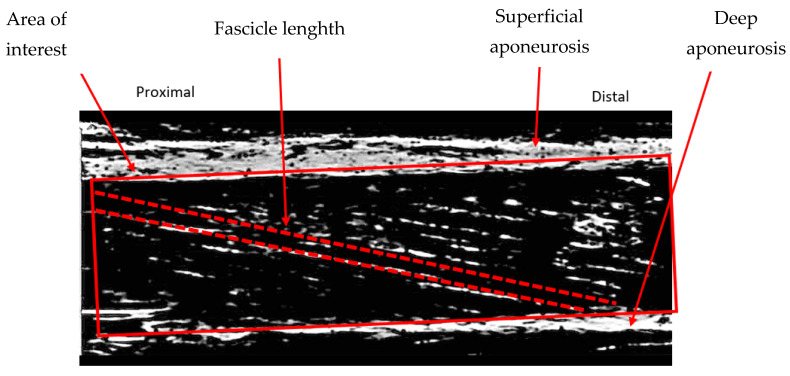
An ultrasound image of the VL of a CP participant.
